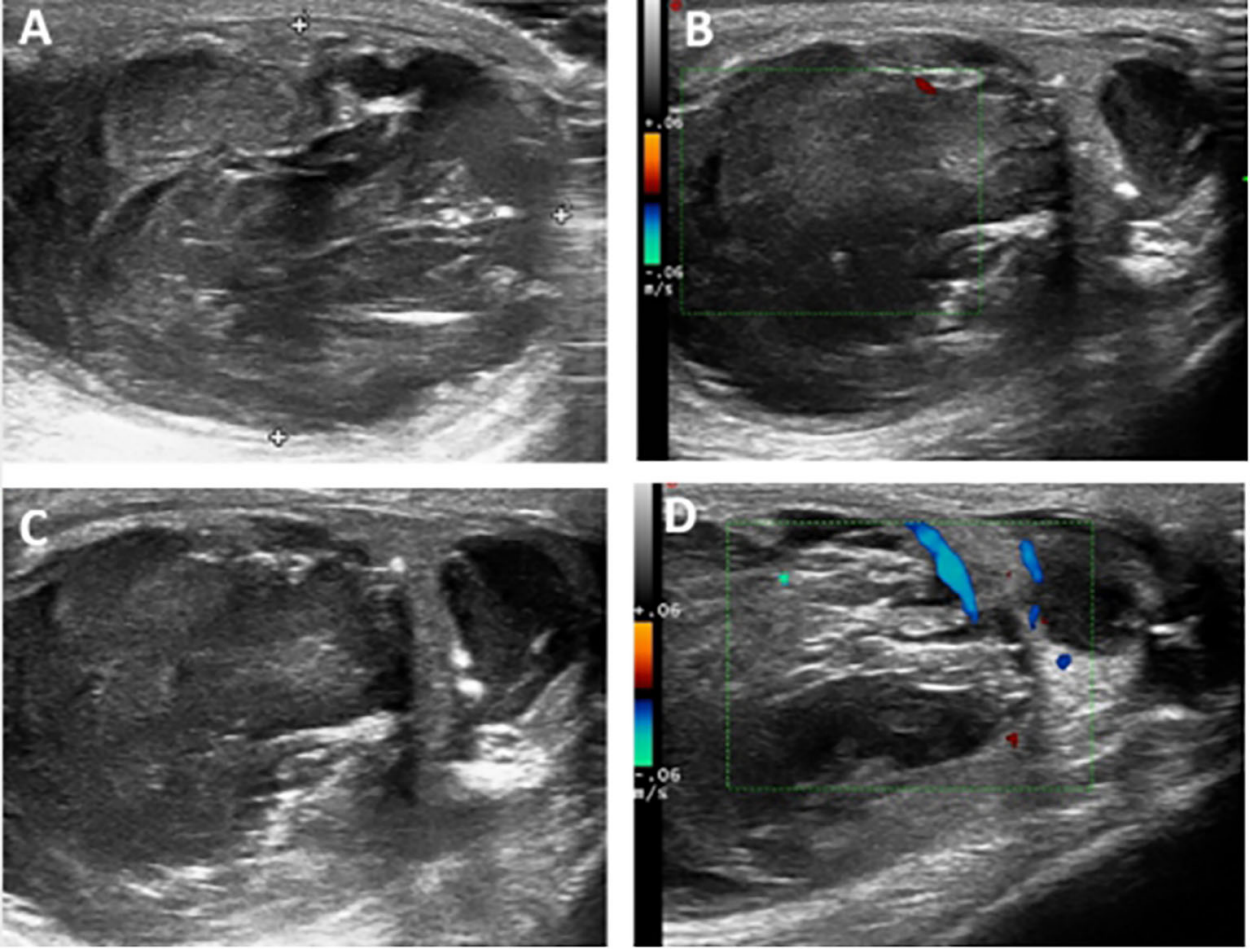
(A) Ultrasound examination of the scrotum revealed a mainly cystic, heterogeneous echogenic mass occupying most of the left testicle. The tumor had a clear boundary, regular morphology, and disordered internal echo. (B, C) Viscous fluid was seen in the cystic part, and large calcified spots were detected in some areas. (D) A few irregular solid protrusions were visible on the cyst wall with a small blood supply.
